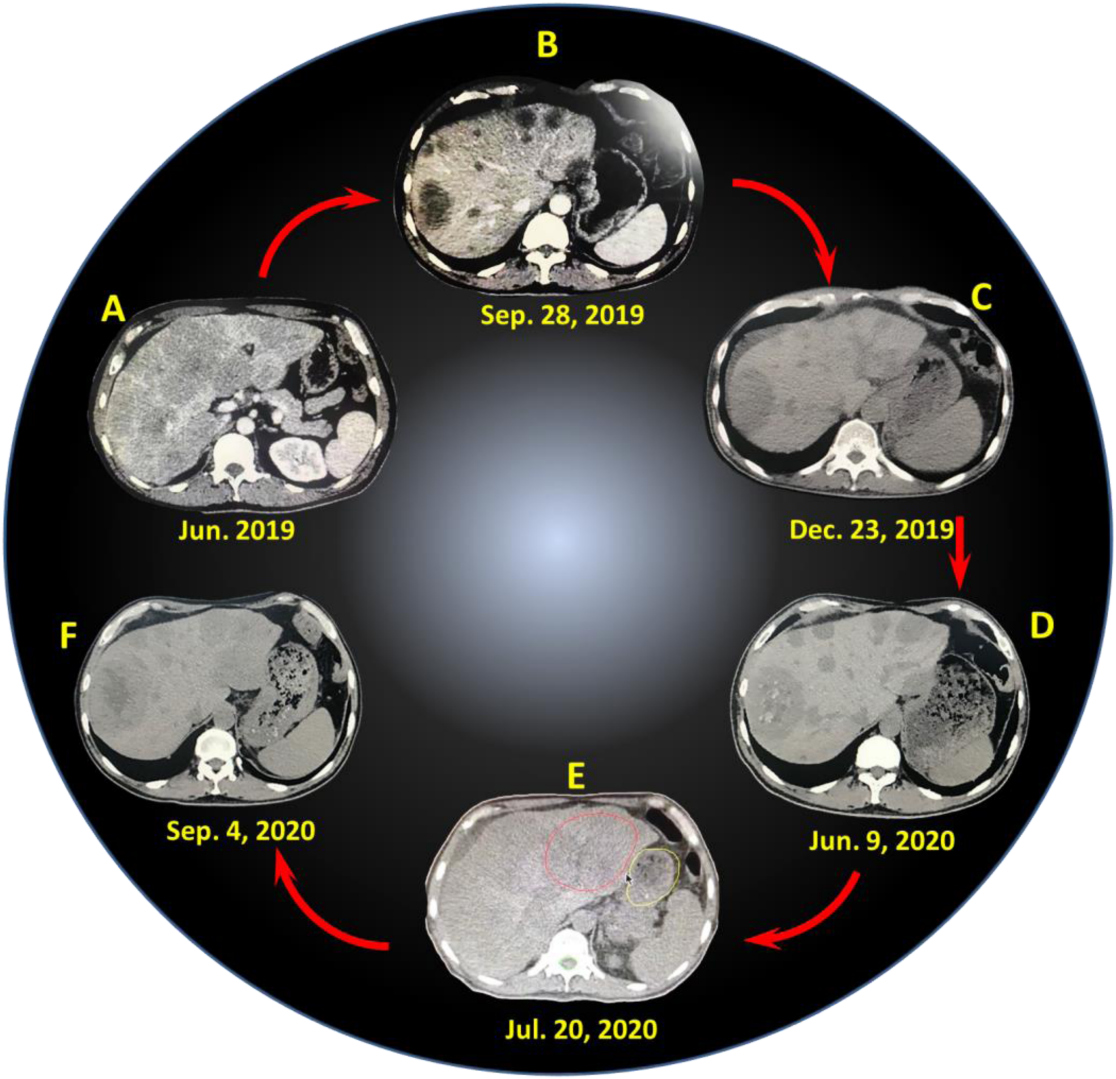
CT images of liver lesions at different time points: (A) June 2019 (before treatment), (B) September 28, 2019, (C) December 23, 2019, (D) June 9, 2020, (E) July 20, 2020, (F) September 4, 2020.
